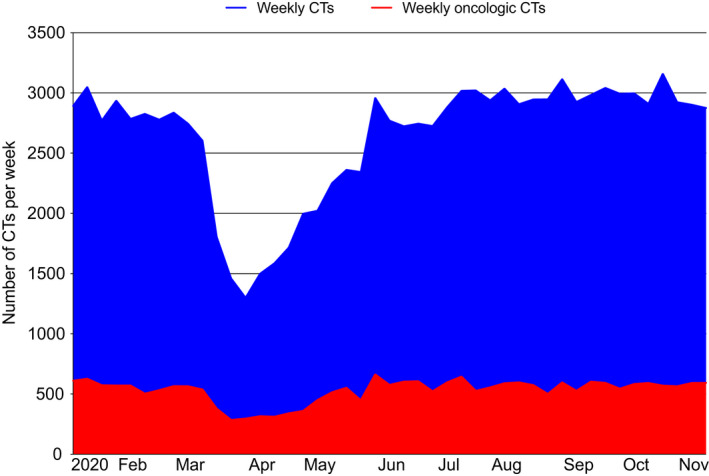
Weekly volumes of all computed tomography (CTs) and oncologic CTs from 5 January 2020 to 14 November 2020
